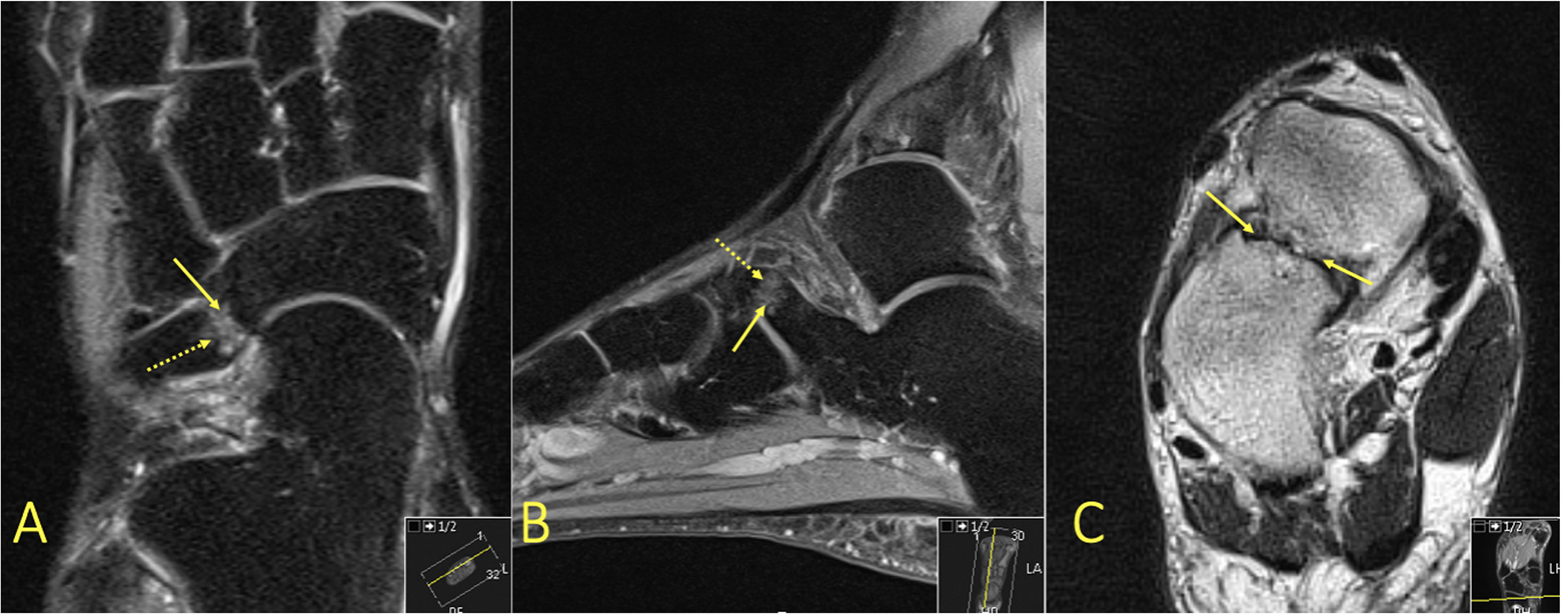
A 51-year-old female with diffuse pain in the ankle and Chopart joints with suspected osteoarthritis. MRI showed a calcaneonavicular coalition (arrows) with adjacent BME (dashed arrow)
